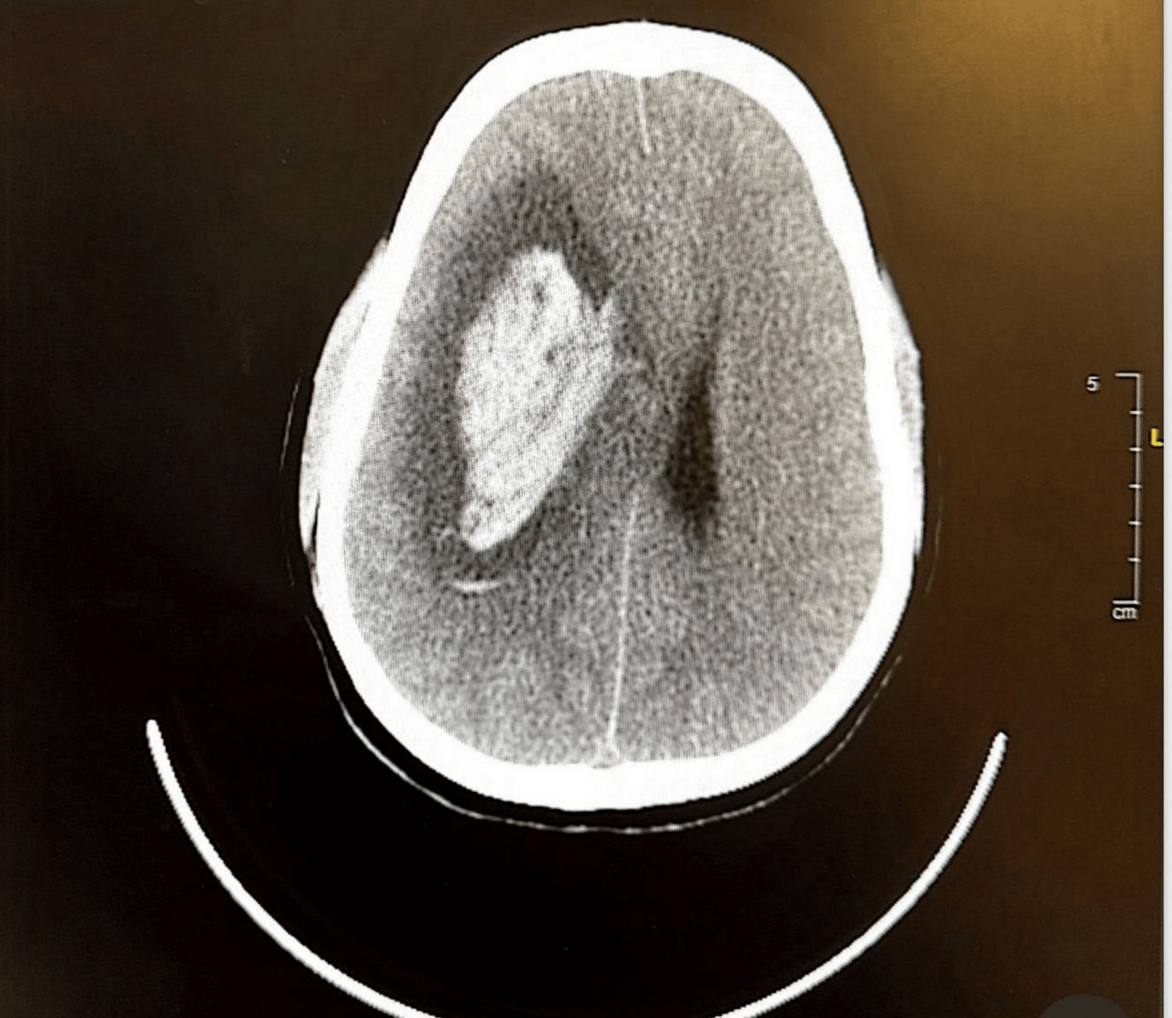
CT of the brain at the time of presentation
